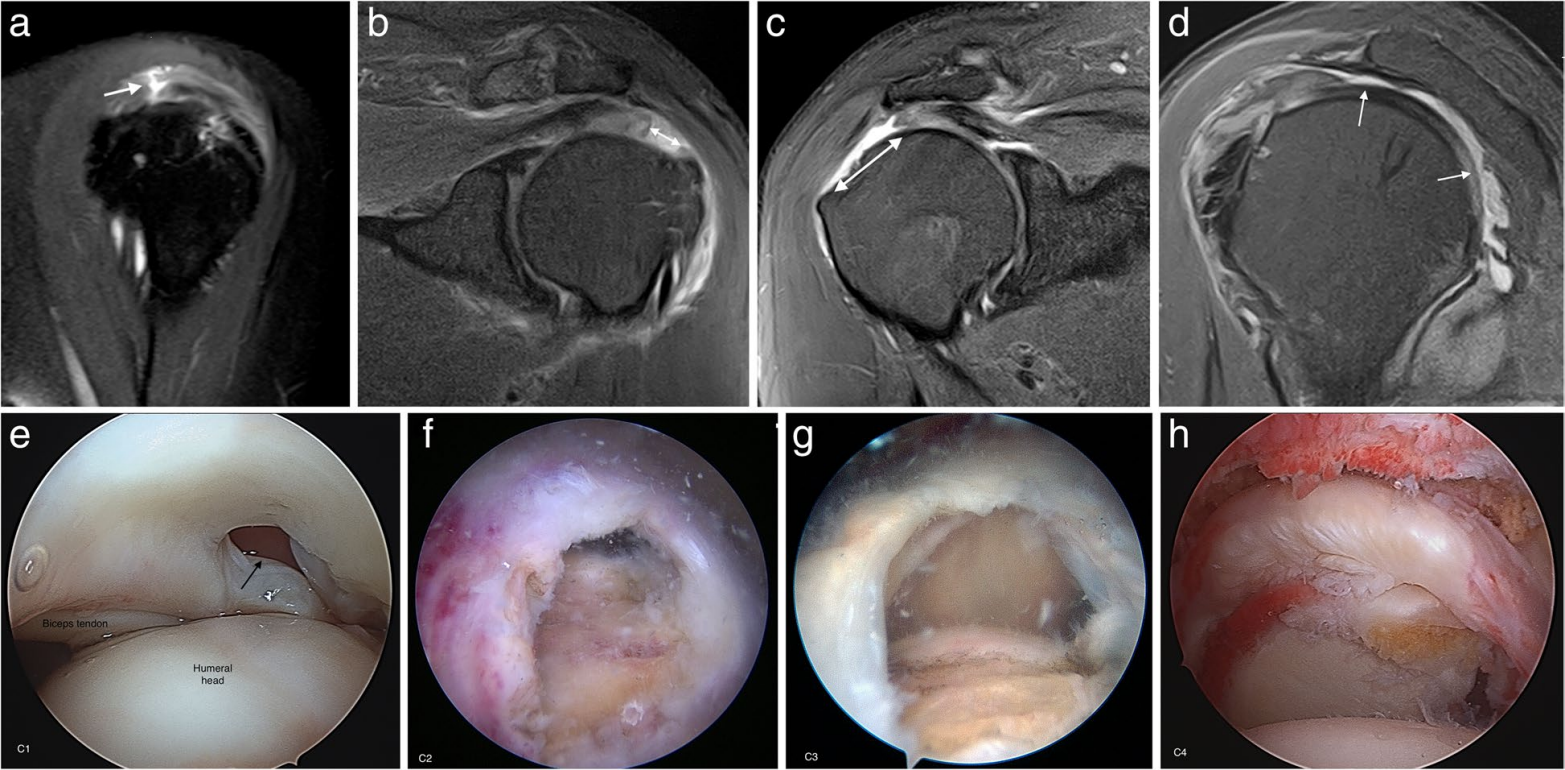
Full thickness tear extension. a Oblique sagittal fat-suppressed PD-weighted MR arthrography showing a C1 tear consisting in a small full thickness tear or pinhole (arrow). b Oblique coronal fat-suppressed PD-weighted MR demonstrates a C2 tear with a small retraction, less than 2 cm (double-headed arrow). c Oblique coronal and sagittal shows a C3 tear which is a large full thickness tear with a retraction usually between 3 and 4 cm (double-headed arrow) affecting only one tendon. d Oblique sagittal fat-suppressed PD-weighted MRI showing a C4 tear consisting in a complete tear of two or more tendons. In this case, there is a tear of both supraspinatus and infraspinatus (arrows). e Arthroscopic view of a C1 tear from the posterior portal (arrow). f–h Arthroscopic views of C2, C3, and C4 tears from a lateral portal
